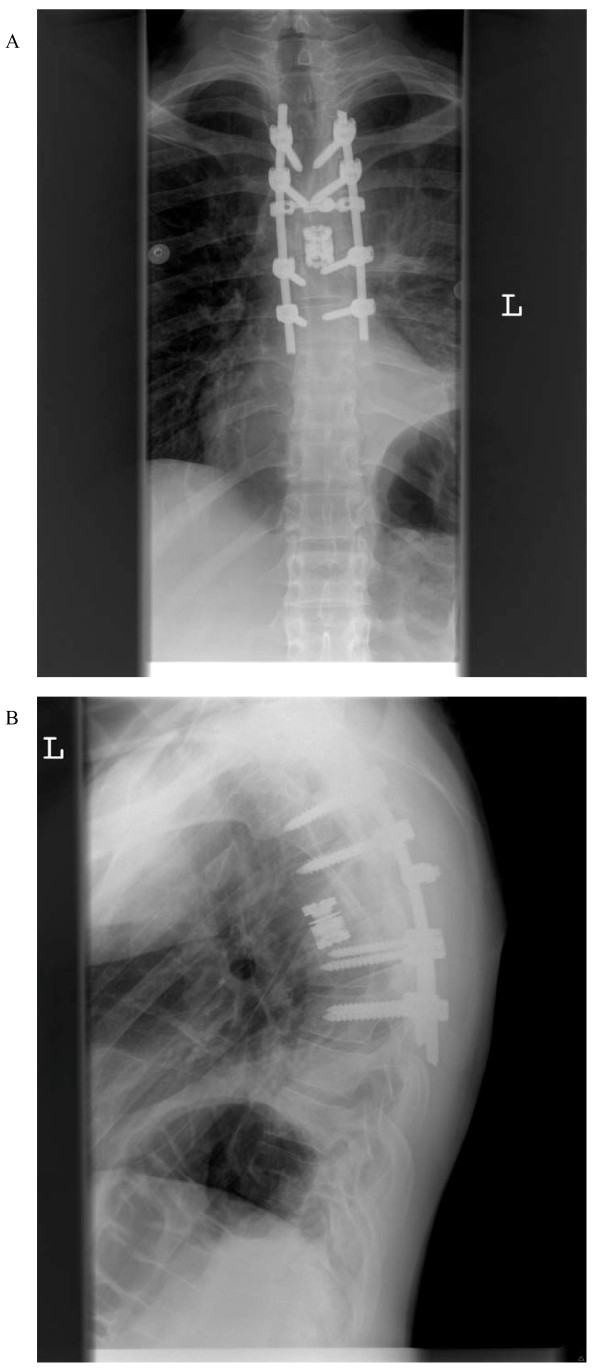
Post-operative standing thoracic spine X-rays (A) AP and (B) lateral shows an expandable titanium cage filling T6 spondylectomy defect and posterior pedicle screw fixation.
